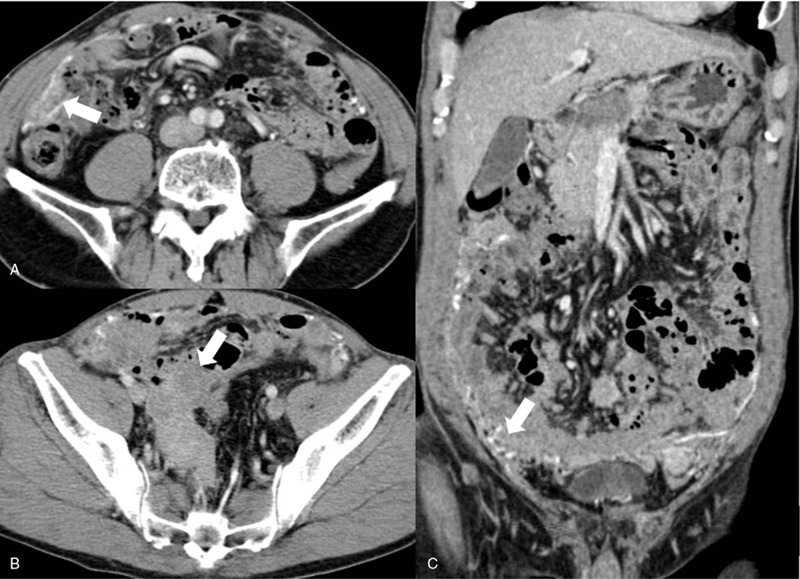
After 5 months treatment of Imatinib, follow-up abdominal CT images (A-C) showed that both pelvic and omental lesions (thick arrow) significantly decreased in size.
